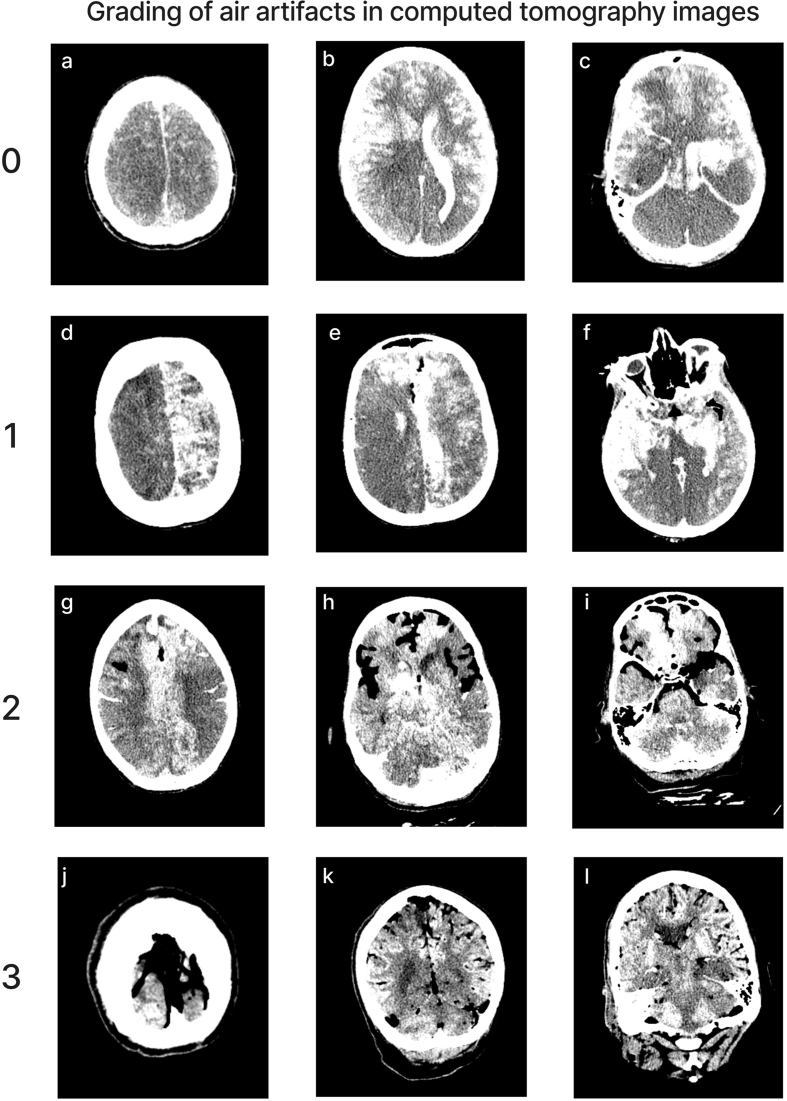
Representative images of CT scans showing the grading of air bubble
